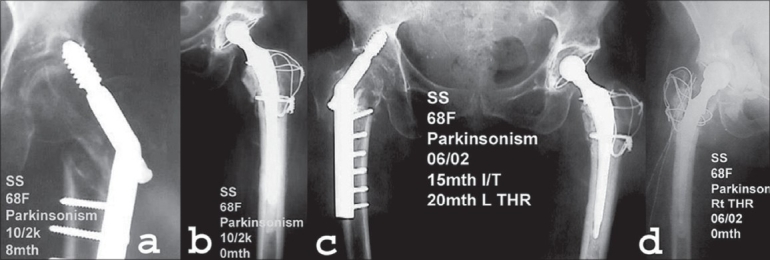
(a) AP radiographs of 68yr old lady with parkinsonism showing lag screw cut out of DHS done for intertrochanteric fracture of left hip done eight months back. (b) DHS was removed and cemented total hip arthroplasty with trochanteric wiring was performed. (c) AP radiograph of same patient showing failure of DHS fixation for intertrochanteric fracture of right hip fifteen months after the fracture. (d) DHS was removed and cemented total hip arthroplasty of right hip was performed
